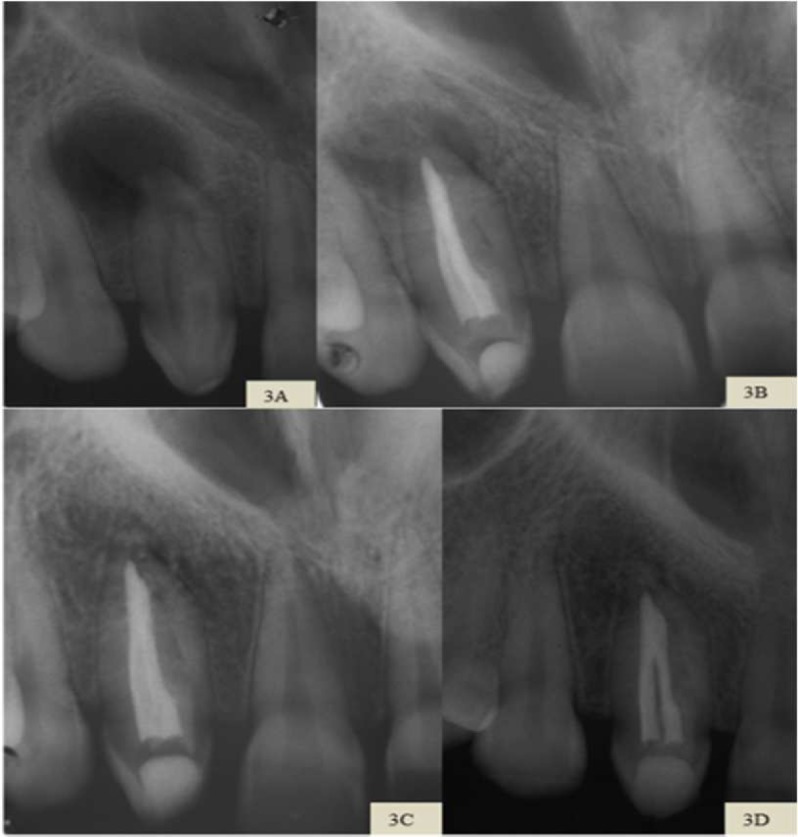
A)* Initial periapical radiography showing bone resorption and presence of invagination in tooth 12; *B)* Final periapical radiography with root canal filling and restoration in tooth 12; *C)* Periapical follow-up radiography after 1 year and 9 months with reduction of periapical lesion and verification of bone neoformation; *D)* After 3 years; demonstrating the normality of the periapical tissues
